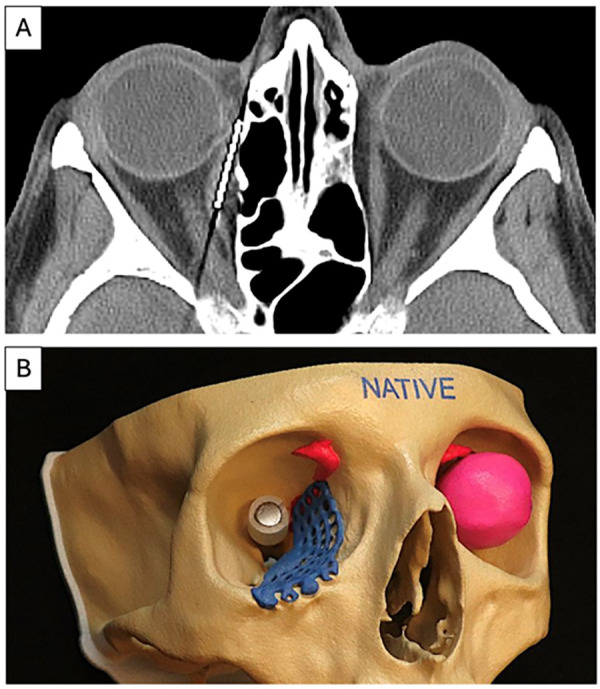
3D printed model to improve safety of hardware removal. (A) Axial soft-tissue CT shows direct contact of the implant with the medial rectus muscle and proximity of the implant edge to the optic nerve. (B) 3D printed model displays the malpositioned orbital implant and its relationship to the globe, optic nerve and superior oblique muscle (globe model was removed from magnetic attachment); surgeons utilize this model for planning of safest vectors for implant mobilization and removal.
